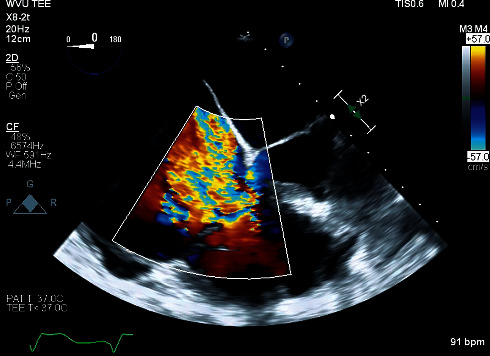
Transesophageal echocardiography, midesophageal four-chamber view demonstrating severe tricuspid regurgitation in patient with a leadless pacemaker.
